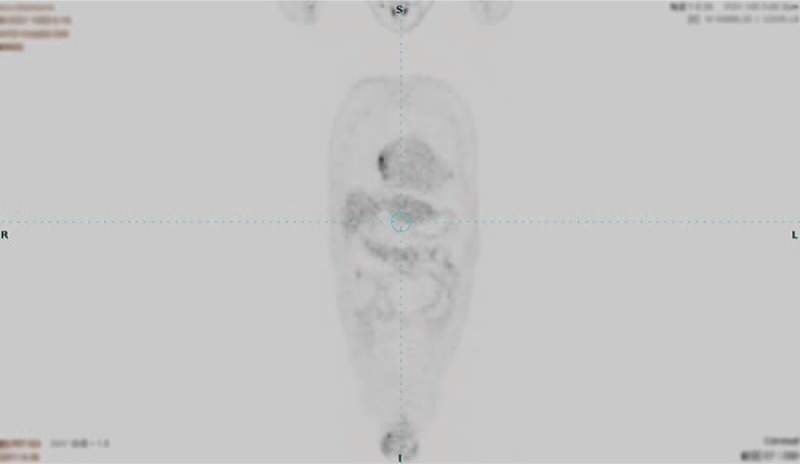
Positron emission tomography–computed tomography demonstrated increased glucose metabolism in right side of the epicardium.
